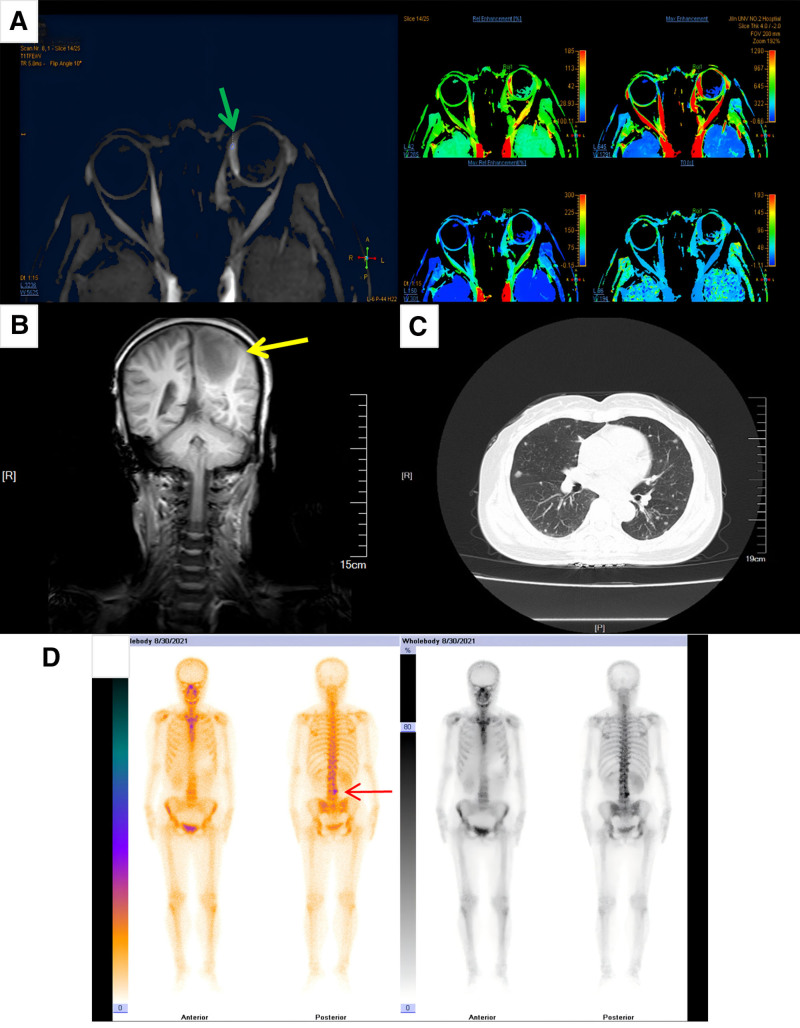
Multiple examinations showed malignancies in the orbit, brain, lung and bone. MRI presented a nasal choroidal tumor (A, green arrow) and an intracranial mass in the left parietal lobe (B, yellow arrow). Chest CT demonstrated multiple pulmonary nodules (C). Whole-body bone scintigraphy with 99mTc-MDP showed increased radiotracer uptake in the L4 vertebra (D, red arrow). CT = computed tomography, MRI = magnetic resonance imaging.
